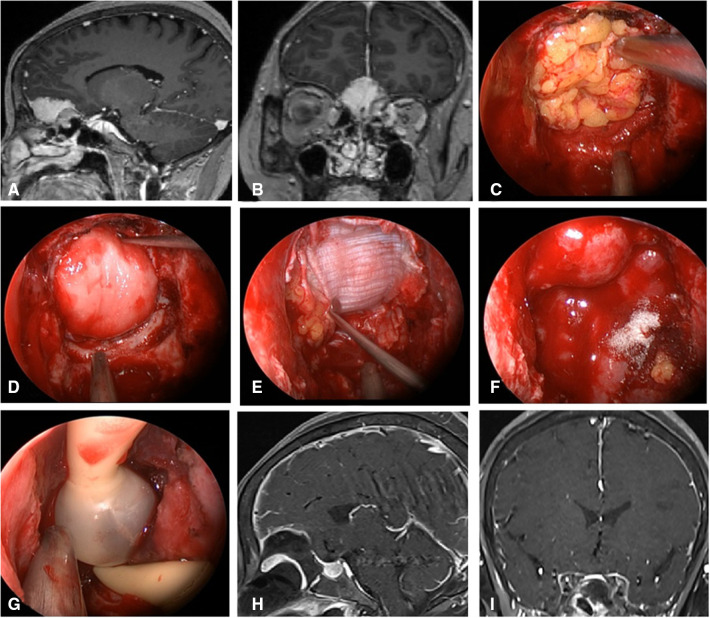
Changes in reconstruction strategy/(before). (A,B) Sagittal and coronal enhanced MRI in sellar region before operation, and olfactory groove meningioma was considered. (C) Subdural fat packing. (D) Artificial dura mater embedded between cellulite and dura mater. (E) Cover the fascia lata on the artificial dura mater. (F) Cover the VP-NSF on the fascia lata. (G) Balloon support. (H,I) Sagittal and coronal enhanced MRI in sellar region after operation, and postoperative pathology showed meningioma.
